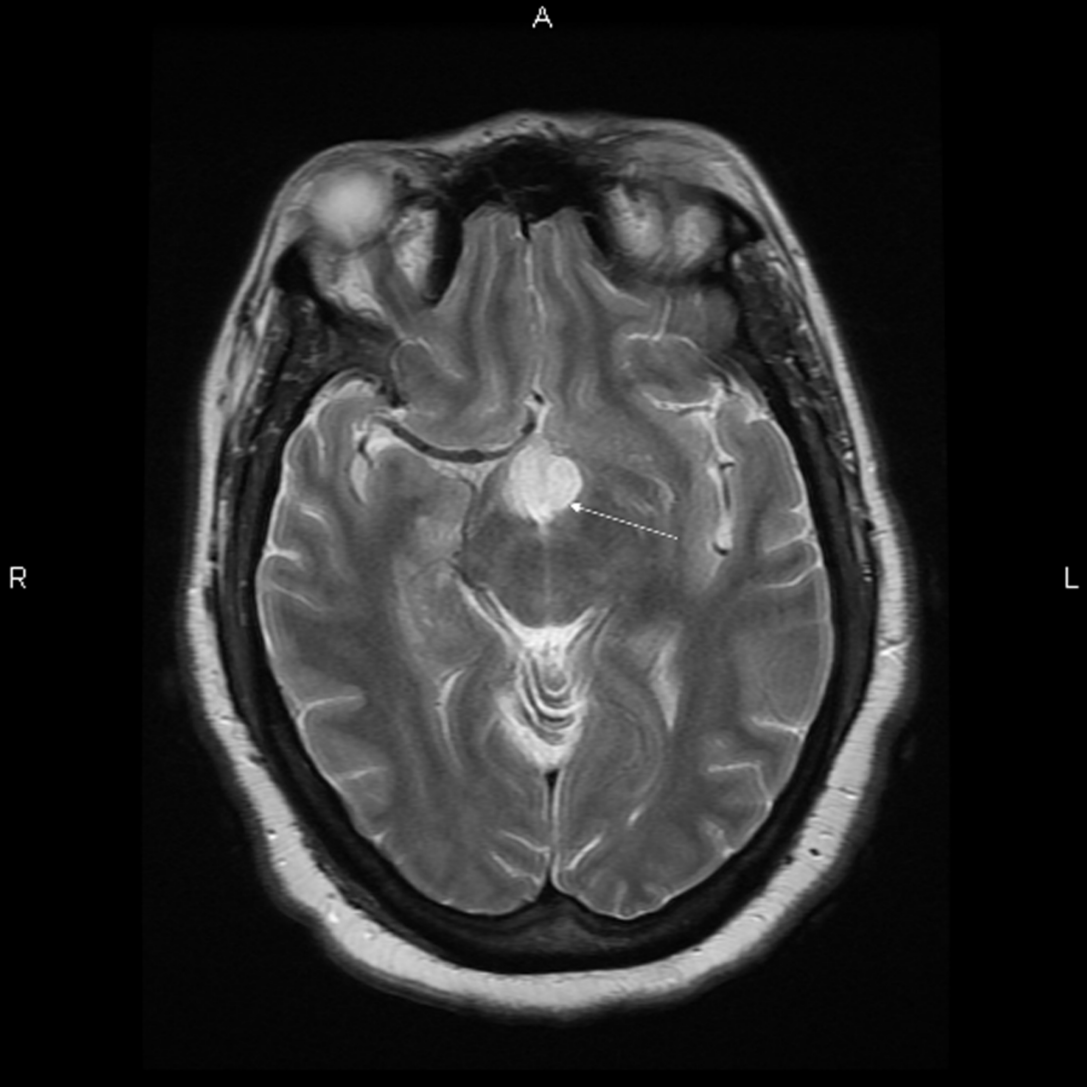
Axial T2 Preoperative brain MRI showing a multi-locular suprasellar cyst (solid white arrow) measuring approximately 1.6 x 1.8 x 2.4 cm
